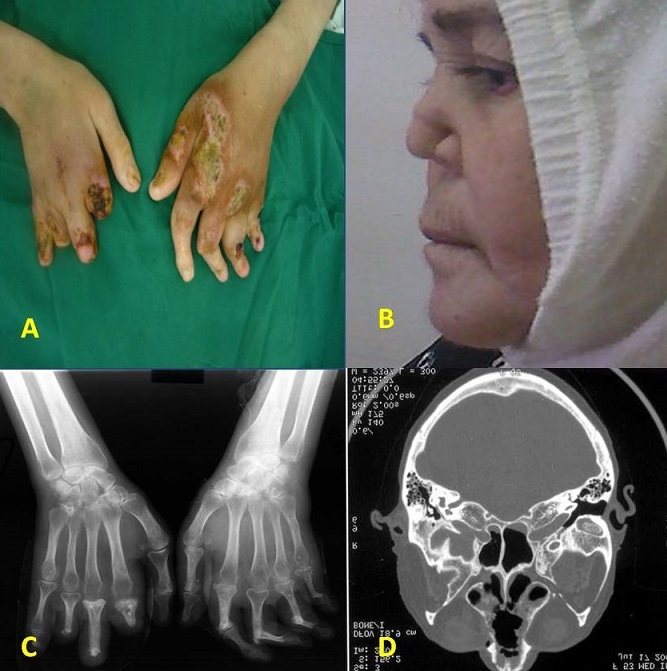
A)atteinte déformante des mains et présence de lésions ulcéro-nécrotiques de la main gauche; B)Effondrement de la pyramide nasale; C)radiographie des mains : une atteinte destructrice et des images d'acro-ostéolyse de certaines phalanges des doigts; D)Scanner du massif facial : Ostéolyse de la cloison nasale. Comblement bilatéral du sinus maxillaire et des cellules ethmoïdales antérieures
